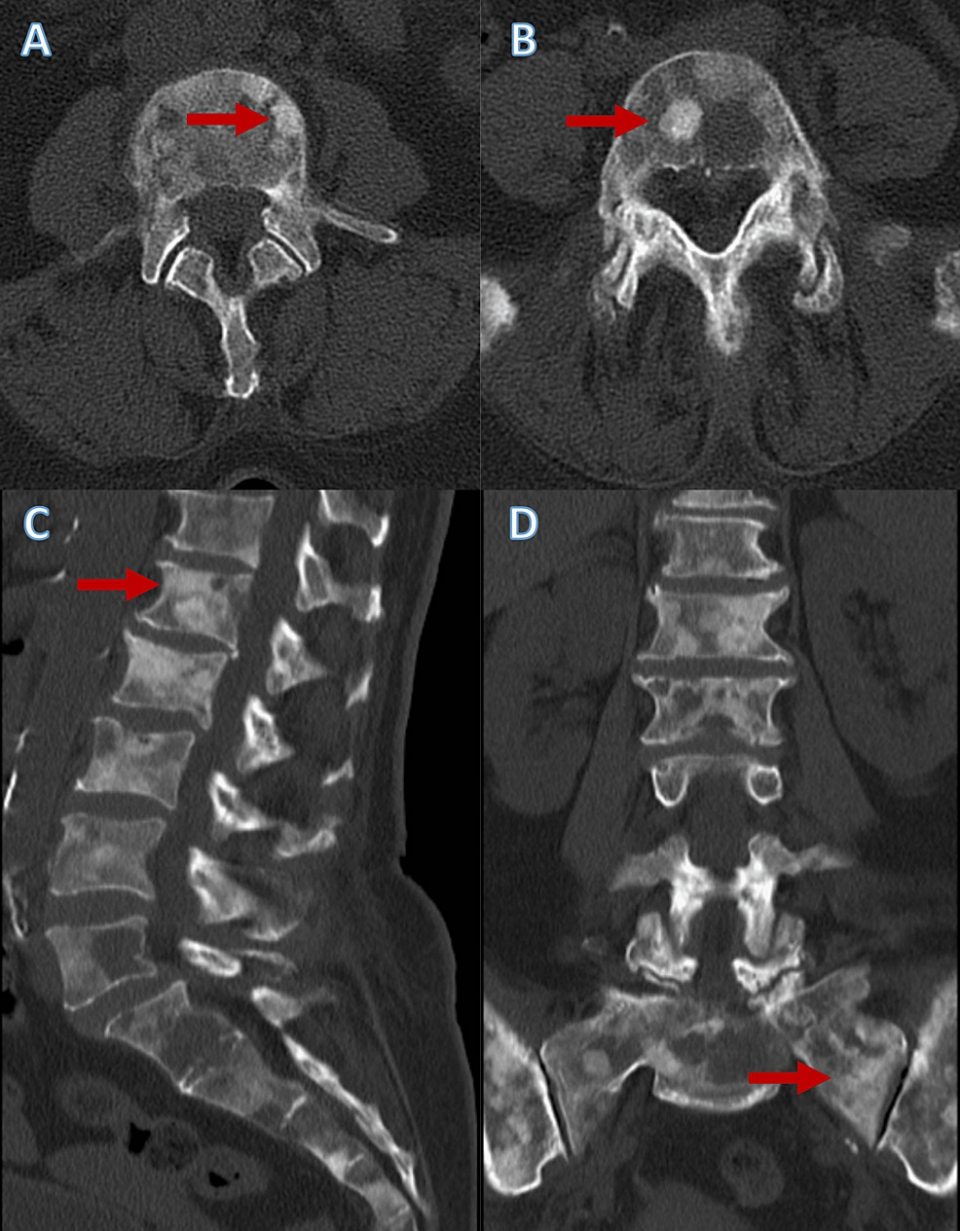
Suspicious vertebral bone lesions Lumbar computed tomography revealed diffuse heterogenety of the lumbar vertebrae and sacrum bone marrow (A, B, C, and D), secondary to malignant infiltration (examples highlighted by the red arrows)
